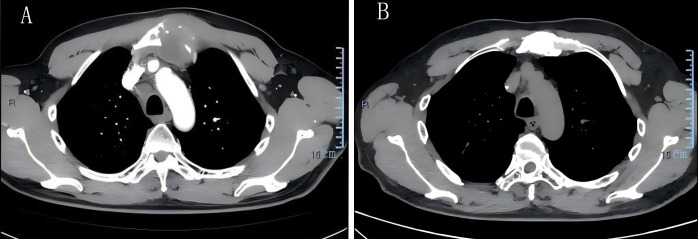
Computed tomography (CT) images of the sternal metastasis. (A) Initial lesion at the manubrium of the sternum. (B) Significant shrinkage of the lesion on January 16, 2026.
